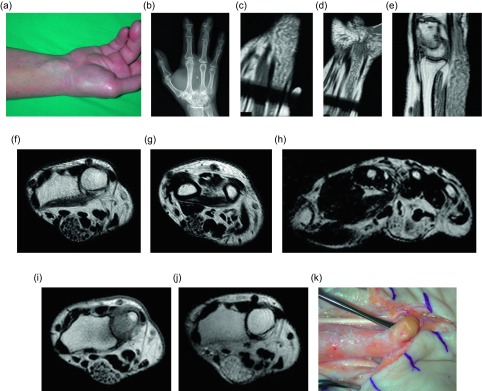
Fibrolipomatous hamartoma: 51-year-old female with prior middle finger resection for congenital macrodactyly, presented with three months of numbness and tingling in the fingers. On physical examination (a), a protruding mass was seen along the course of the median nerve at the carpal tunnel. Corresponding frontal radiograph (b) of the left hand demonstrates postoperative changes from resection of the middle finger with heterotopic bone formation in the surgical bed. Advanced degenerative changes are seen, predominately at the thumb carpometacarpal and distal interphalangeal joints. Coronal (c, d) and sagittal T1W (e) images of the left wrist demonstrate the serpentine appearance of the median nerve fascicles with surrounding fat proliferation. Axial T2W non-fat-saturated images of the left wrist reveal fibrofatty infiltration of the median nerve (f, g). These images demonstrate the “co-axial cable” appearance of the nerve fascicles. The digital nerve branches are also involved (h). Pre- (i) and post-contrast (j) axial T1W images of the left wrist show avid enhancement of the median nerve fascicles following the administration of intravenous gadolinium. Intraoperative image (k) shows a fibrofatty mass of the median nerve at the distal left wrist, corresponding with mass seen on physical examination.
